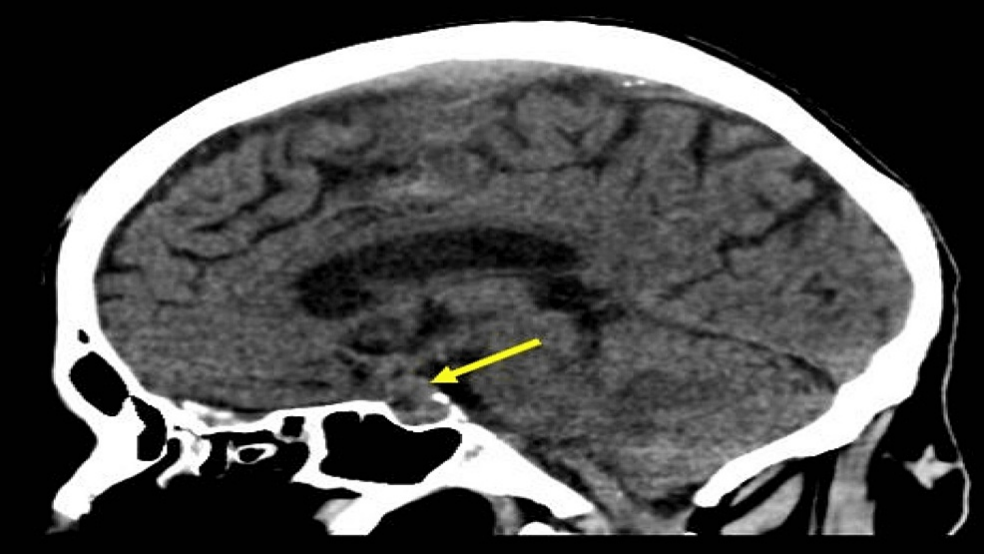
Sagittal non-contrast CT brain shows a well-defined isodense lesion in the pituitary fossa widening of the sella with suprasellar extension (yellow arrow). No frank intracranial hemorrhage was visible.
